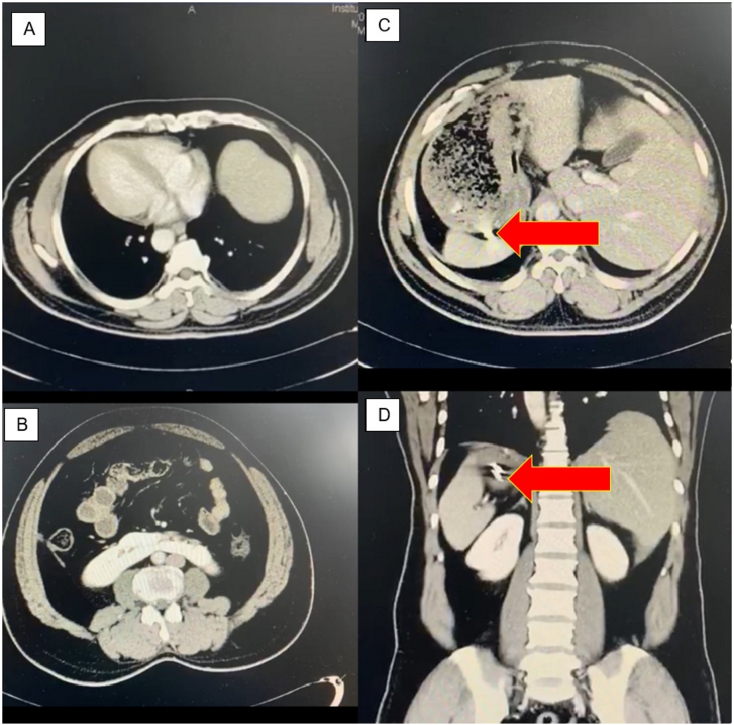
(A) A CT scan of the abdomen at the axial view showing SIT. (B) The duodenum crosses from left to right up to the duodenu-jejunal junction. (C) The foreign body artefacts (red arrow) were seen on a CT scan at the axial view. (D) The similar artefacts (red arrow) at the coronal view of the CT scan.
